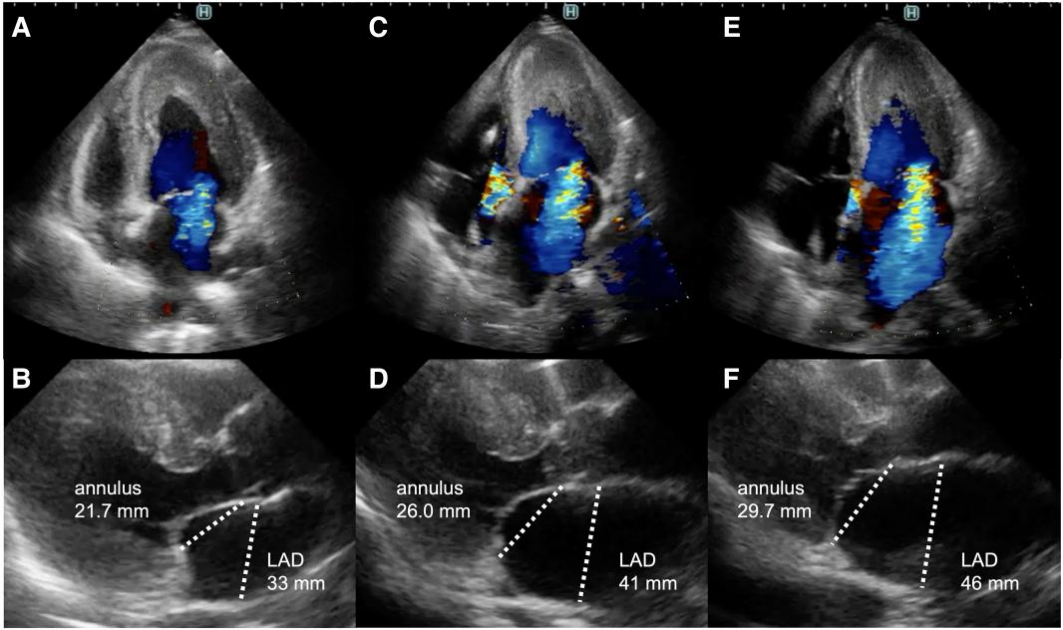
Transthoracic echocardiographic findings. On admission, the mitral valve closure position is seen to be dilated towards the LV apex, primarily due to tethering-related mild MR (*A* and *B*). The left atrium and annulus are enlarged, and the MR is worsened to moderate on day 4 (*C* and *D*). The MR is worsened to severe significantly on day 11 (*E* and *F*). LAD, left atrium dimension; LV, left ventricular; MR, mitral regurgitation.
